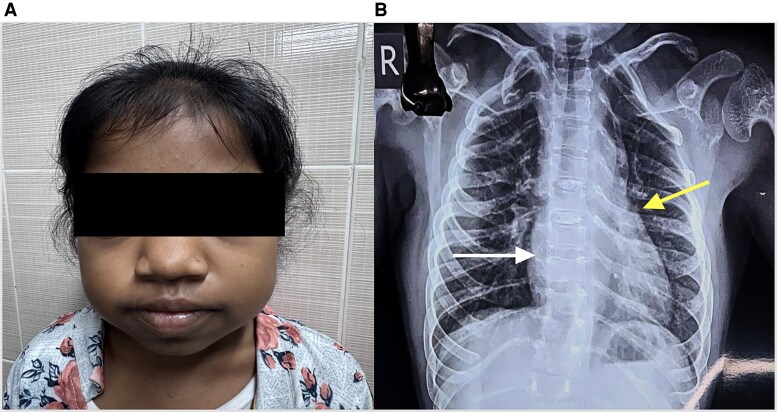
(*A*) Image of the child’s face showing characteristic facial features, such as flattened nasal bridge, short neck, thick lips, wide mouth, and sparse hair. (*B*) Chest X-ray posteroanterior (PA) view demonstrating the double atrial shadow (bottom white arrow) and straightened left heart border (top right arrow).
